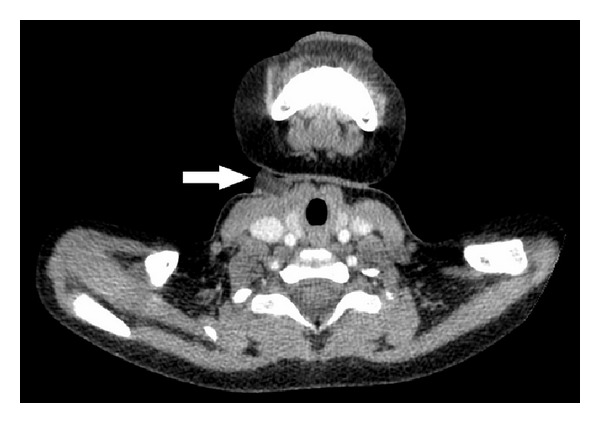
Computed tomography section that shows the foreign body in the right side of the neck (arrow).
